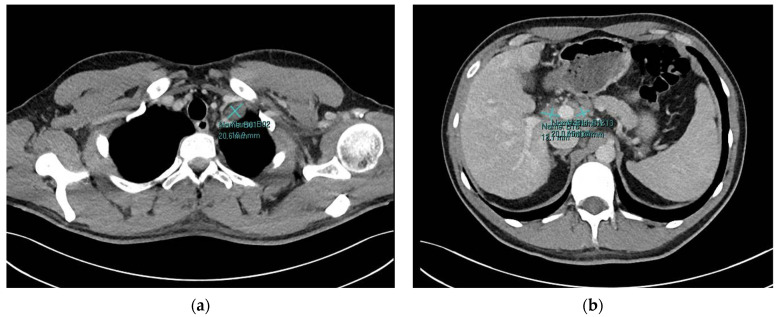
CT scan at relapse showing (a) a supraclavicular lymph node measuring 16 × 20 mm and (b) hepatic hilar lymph nodes measuring 13 × 20 mm and 15 × 10 mm.
